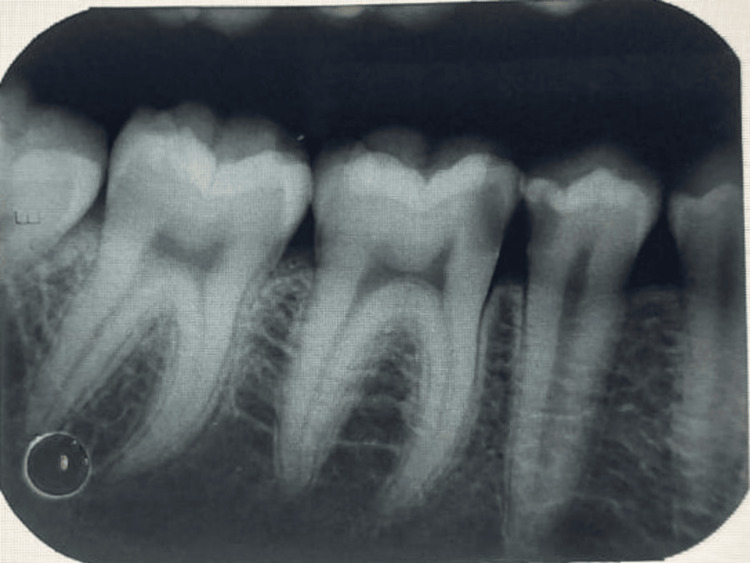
Preoperative intraoral periapical radiograph of the mandibular right first molar tooth
